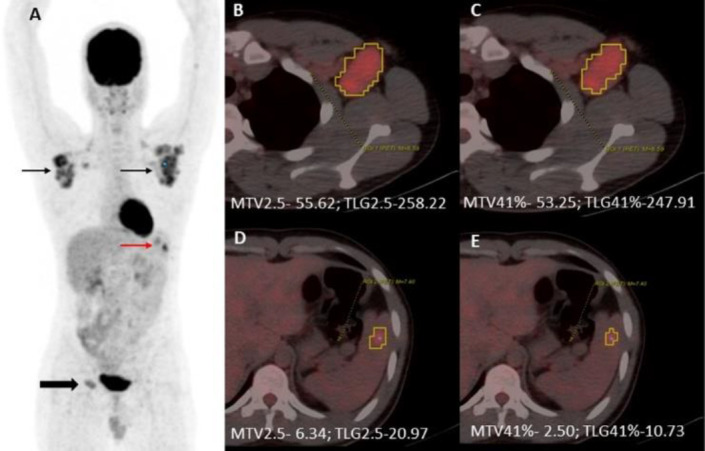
MTV and TLG calculation in a representative patient with advanced stage Hodgkin Lymphoma. (A) MIP image depicts FDG avid bilateral axillary (black arrows), inguinal (block black arrow) adenopathy, along with splenic lesion (red arrow). Fused PET/CT images depict MTV and TLG calculation of the left axillary node with a fixed cut-off of 2.5 (B) and percentage cut-off of 41% (C). Again, fused PET/CT images are depicting MTV and TLG determination of focal splenic lesion with a fixed cut-off of 2.5 (D) and a percentage cut-off of 41% (E). Abbreviations: MIP, Maximum Intensity Projection; FDG, Fluorodeoxyglucose; PET/CT Positron Emission Tomography/ Computed Tomography; MTV, Metabolic Tumor Volume; TLG, Total Lesion Glycolysis
